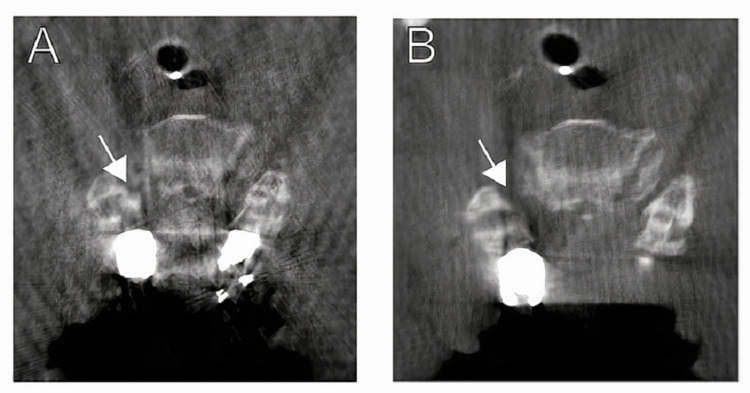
Case 2 – Malpositioned C4/5 interfacet implant with intraoperative removal The intraoperative CT scan demonstrates a medialized right C4/5 interfacet spacer causing impingement of the neural foramen (A). The malposition was recognized and the interfacet spacer was removed. A second intraoperative CT scan demonstrated resolution of foraminal stenosis at that level (B).
